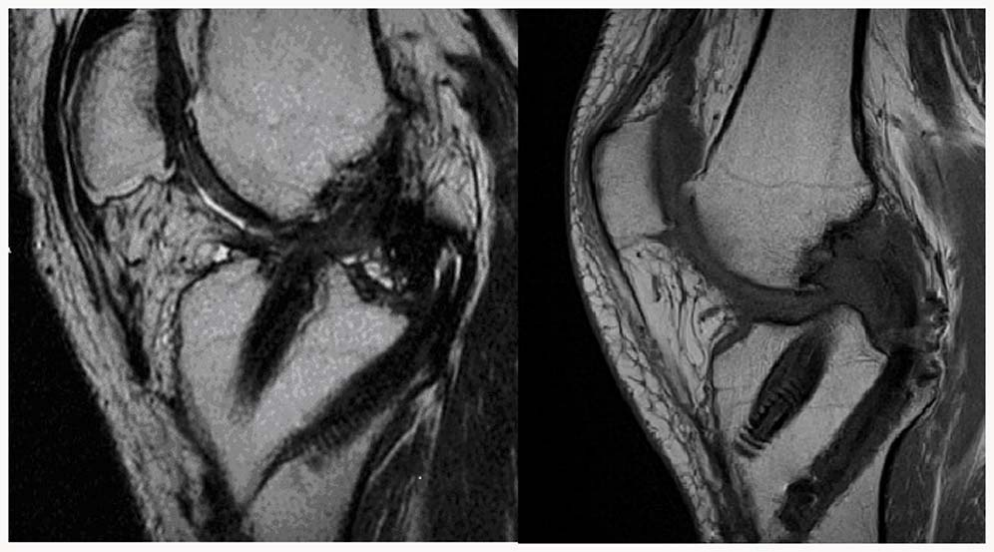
MRI of a knee 5 years after a simultaneous arthroscopic reconstruction of the ACL and the PCL with hamstring tendons showing an proper localization and structure of grafts without knee subluxation.
